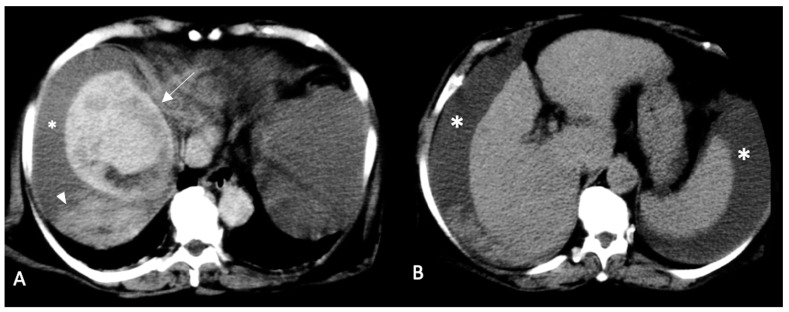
Multi-phase enhanced CT scan (portal phase (A) and late phase (B)). Large HCC of VII-VIII segment with intracapsular hemorrhage and sentinel clot (arrow). Peri-hepatic and peri-splenic ascites effusions are also visible (asterisks). Perihepatic fluid appears to be contaminated by blood in the dependent site (hematocrit sign, arrowhead (A)).
